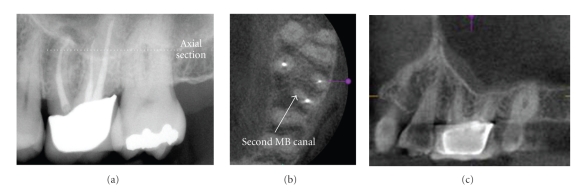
Maxillary first and second molars often present treatment challenges because of the frequent presence of mesioaccessory (mb2) canals. On initial periapical radiographic examination of this previously treated maxillary left first molar, no mb2 canal was detected; however a periapical lesion is seen (a). Note the overlap of the interproximal contacts between the molars indicating a geometric distortion in the horizontal plane. CBCT imaging ((b) 0.076 mm axial and (c) 0.076 parasagittal) clearly demonstrates an additional canal that was not previously treated.
